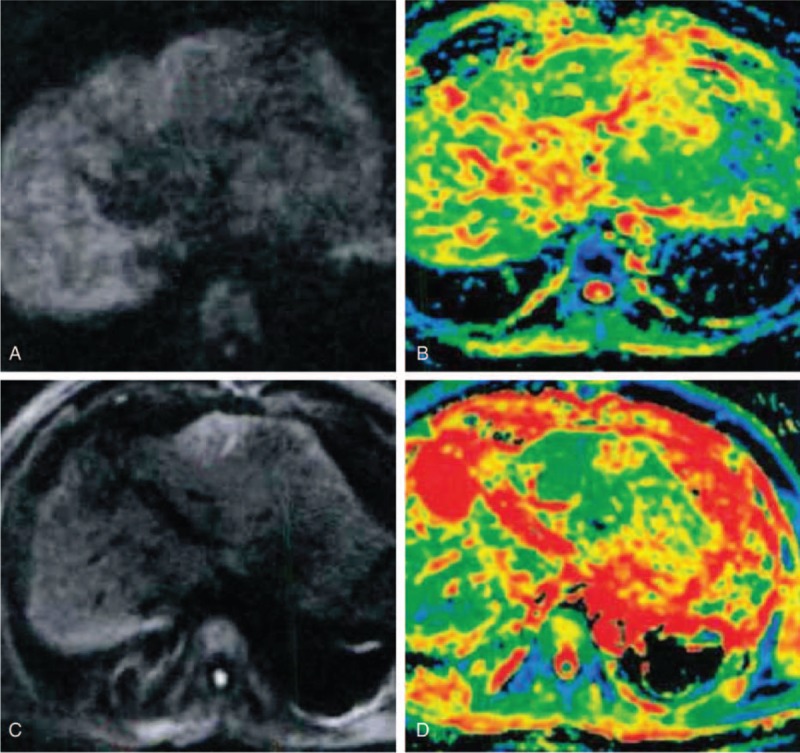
MR-DWI images of HCC patients before and after TACE combined with CT-guided RFA treatment. (A) Homogeneous high MR-DWI signal before 1 day of TACE; (B) significant low signal by ADC before 1 day of TACE; (C) significant decreased MR-DWI signal of the lesion after 1 month of RFA; and (D) significant increased ADC signal of the lesion after 1 month of RFA. ADC = apparent diffusion coefficient, CT = computed tomography, MR-DWI = diffusion weighted magnetic resonance imaging, HCC = hepatocellular carcinoma, RFA = radiofrequency ablation, TACE = transcatheter arterial chemoembolization.
